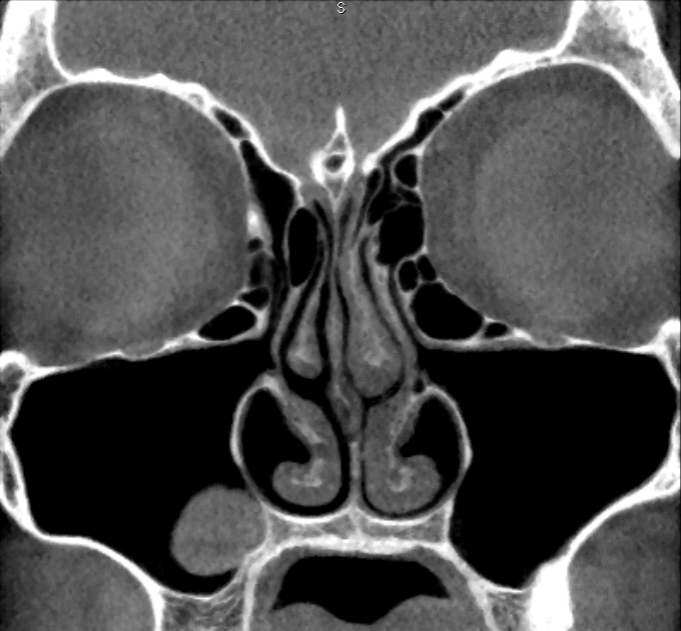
Bilateral Haller cells on coronal plane of CBCT
